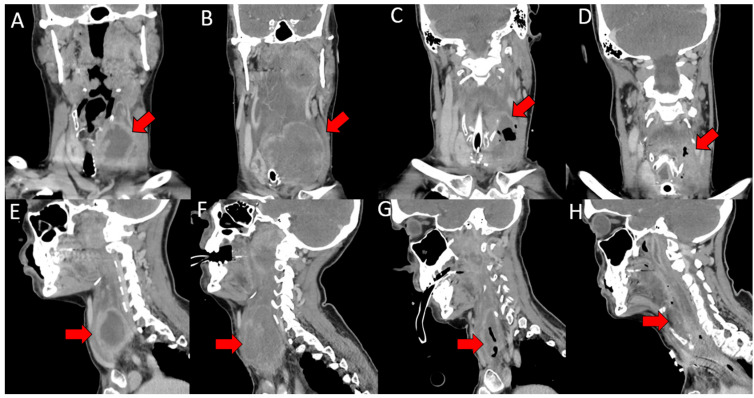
Computed tomography (CT) neck images of the patient demonstrating the size changes in the primary tumour; the tumour is indicated with the red arrow in all images. Images (A–D) are coronal plane images and images (E–H) are sagittal plane images. (A,E): Earliest CT imaging, at initial presentation, showing a left-sided neck tumour beginning to displace the trachea, measuring 6.0 × 4.4 × 4.7 cm. (B,F): CT imaging from 2 months post-presentation, with the patient intubated in intensive care, showing the primary tumour enlarged to 8.1 × 7.8 × 5.0 cm. (C,G): CT imaging from 2.5 months post-presentation after 9 days of dabrafenib-trametinib administration, displaying a shrinkage of tumour to 7.1 × 6.3 × 3.8 cm. (D,H): CT imaging from 5 months post-presentation, after 3 months of dabrafenib-trametinib administration, tumour approximately 3.2 × 3.5 × 4.3 cm.
